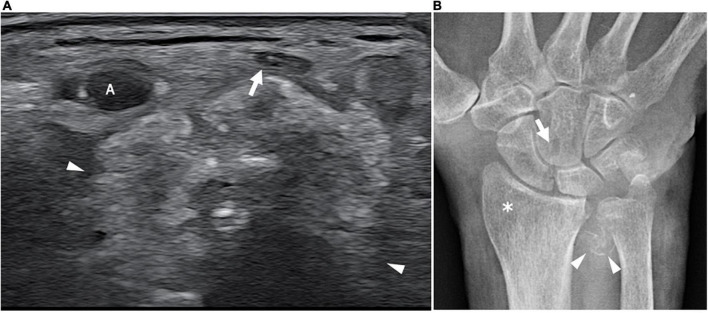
This figure shows an axial 18 MHz HRUS image of the wrist (A) and an antero-posterior X-ray image of the wrist in a 68 years old female affected by systemic sclerosis and monolateral carpal tunnel syndrome. (A) The distal radio-ulnar joint recess is distended by hyperechoic amorphous material partially calcified (white arrowheads) which compresses and superficially dislocates the median nerve (white arrow). (B) The X-ray confirms the presence of calcification (white arrowheads) in correspondence to the soft tissue in proximity to the distal radio-ulnar joint. Para-articular osteoporosis (white asterisk) and erosions (white arrow) at the level of the carpal bones are also observed. A, radial artery.
